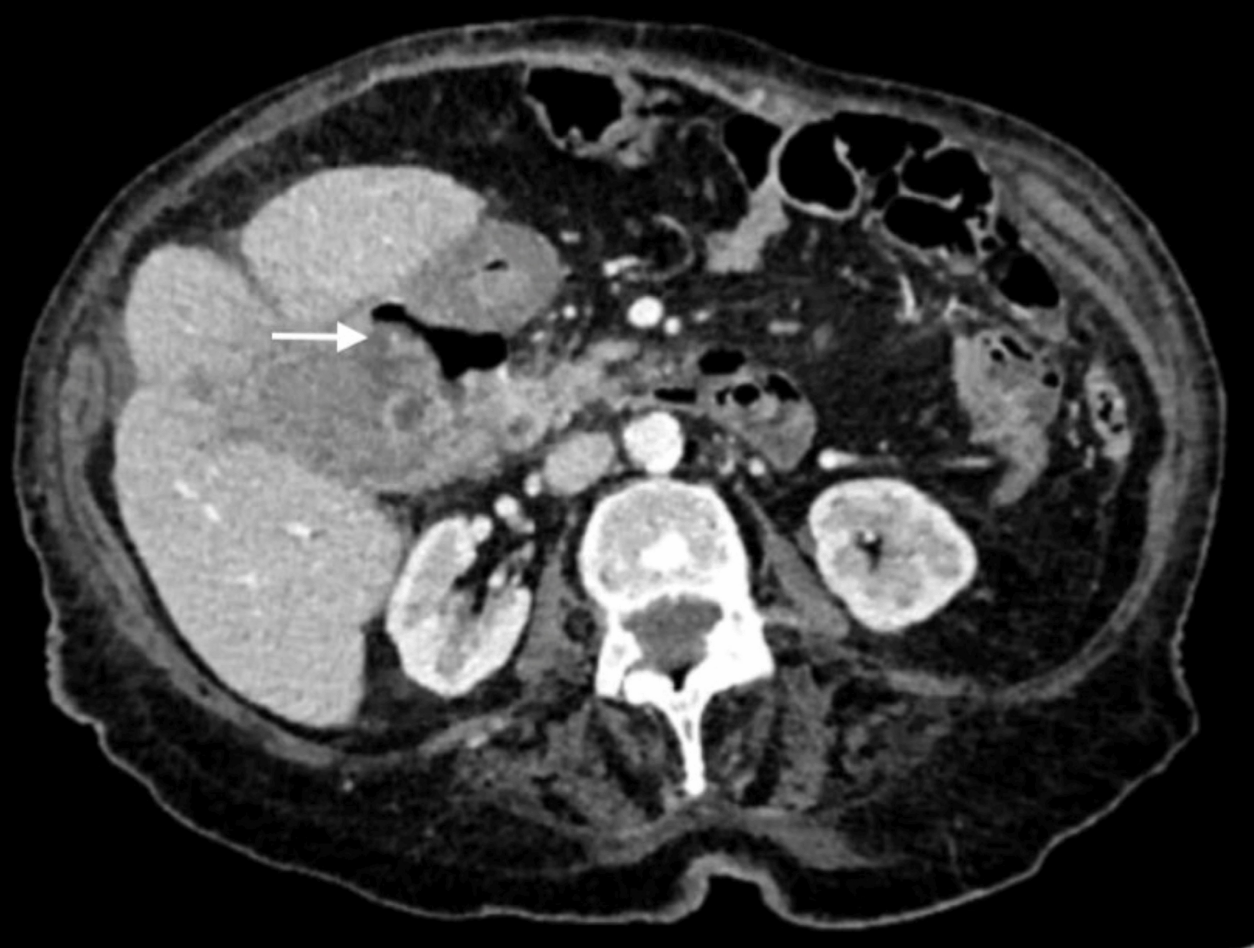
Axial abdominal computed tomography with intravenous contrast shows gallbladder wall thickening with edema. The white arrow indicates the fistulous communication between the gallbladder and the duodenum
